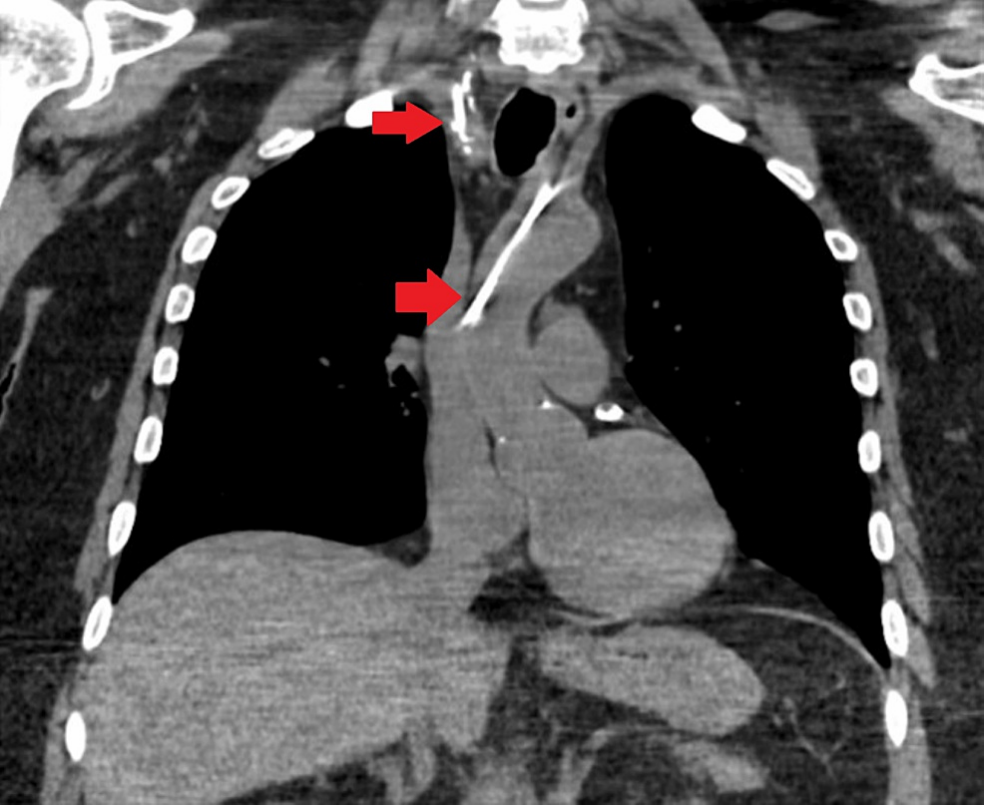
CT chest demonstrating catheter entering subclavian artery and tip terminating in the thoracic aorta
